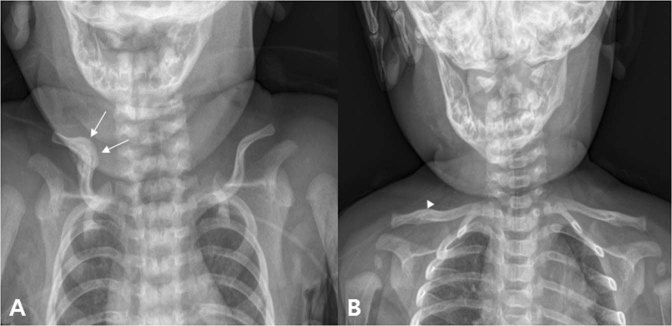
An infant who visited our hospital for left torticollis at 1 month after birth. In ultrasound examination, there was muscular fibromatosis in left SCM. (A) At 1 month after birth, callus formation around the fracture site of right clavicle was seen (arrow). For clavicular fracture, only a conservative treatment was performed except stretching exercise and home education for left CMT. (B) Follow-up plain radiograph at 4 month after birth. Callus formation was nearly disappeared, and it is difficult to find the difference between left and right clavicle (arrowhead).
